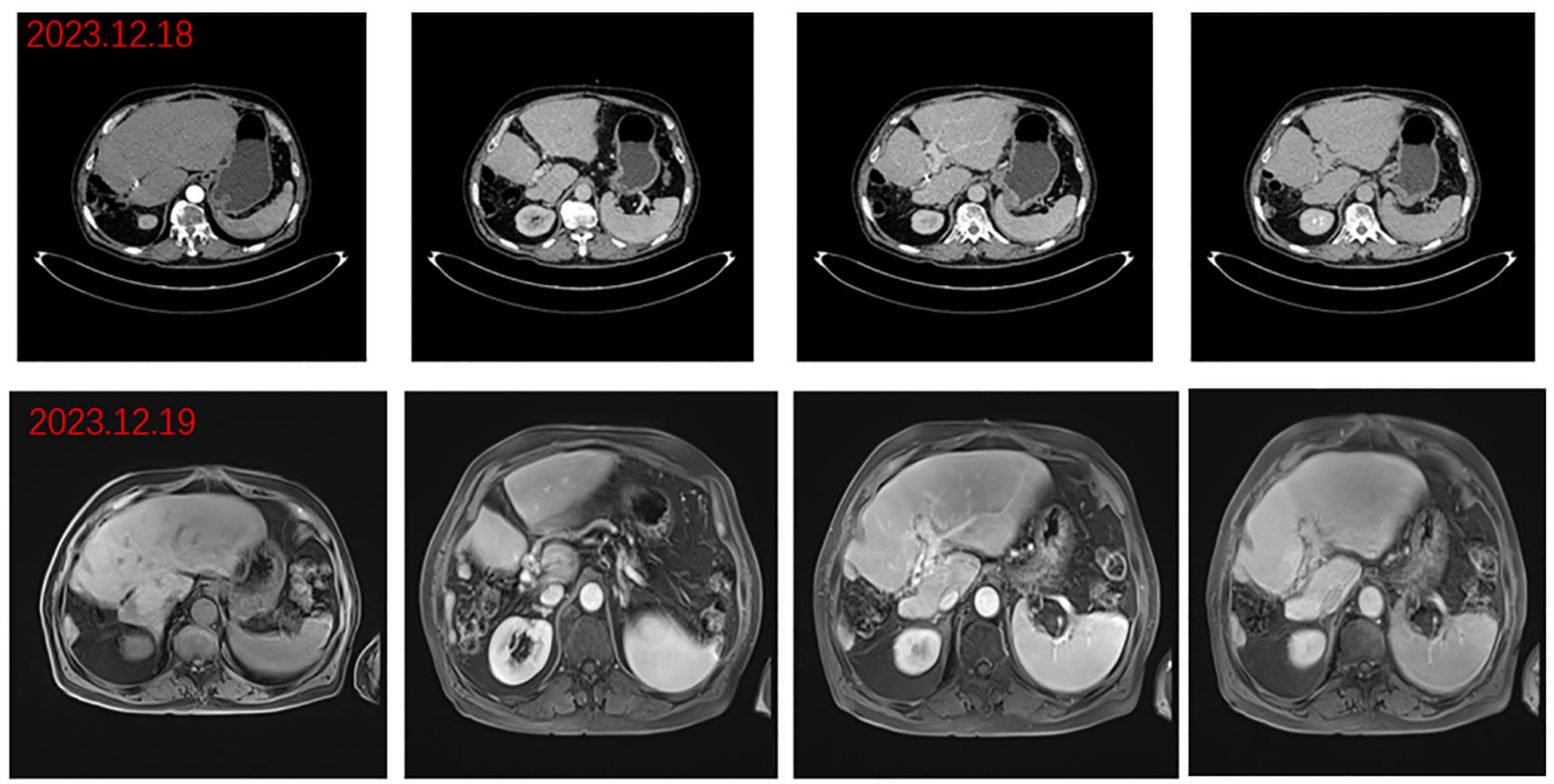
Postoperative images. Arterial, portal, and delayed phase CT: disappearance of portal cancer embolus and resection of right lobe lesion and portal phase, delayed phase abdominal MR.
